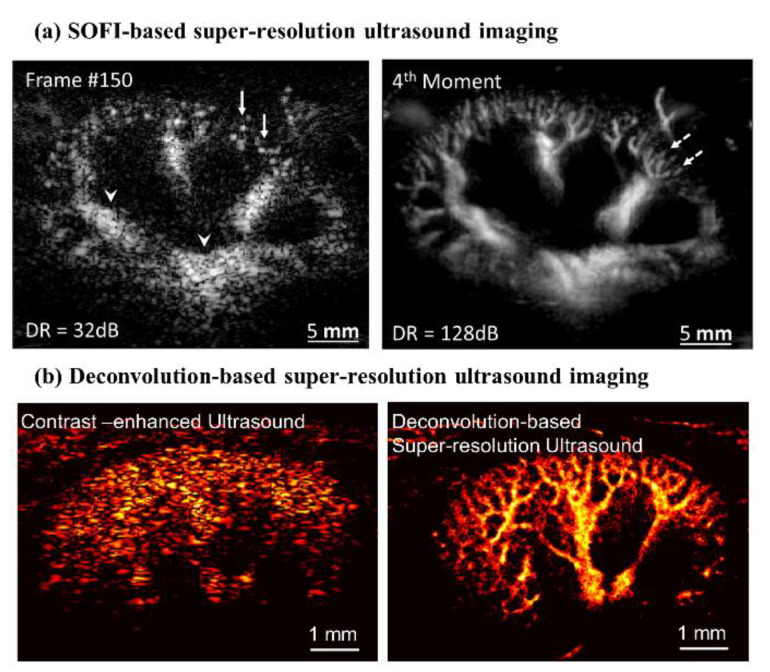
(a) SOFI-based super-resolution ultrasound imaging—left panel shows a frame of B-mode; right panel shows the reconstructed image. (Reprinted with permission from Ref. [43]. Copyright 2017 IEEE). (b) Deconvolution-based super-resolution ultrasound imaging—left panel shows a frame of B-mode; right panel shows the reconstructed image. (Reprinted with permission from Ref. [45]. Copyright 2020 Elsevier).
